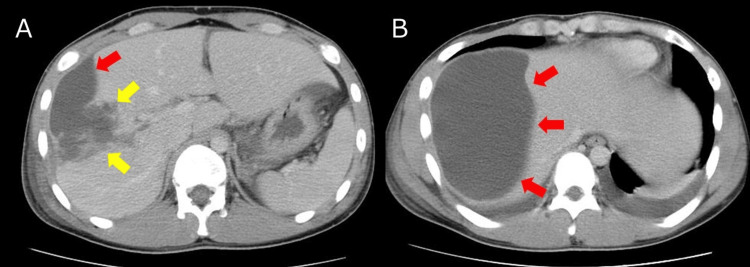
CT on hospital day 14 (biloma) (A) Axial CT showed an encapsulated perihepatic fluid collection adjacent to the laceration (yellow arrows). (B) Large perihepatic fluid consistent with biloma (red arrows).
